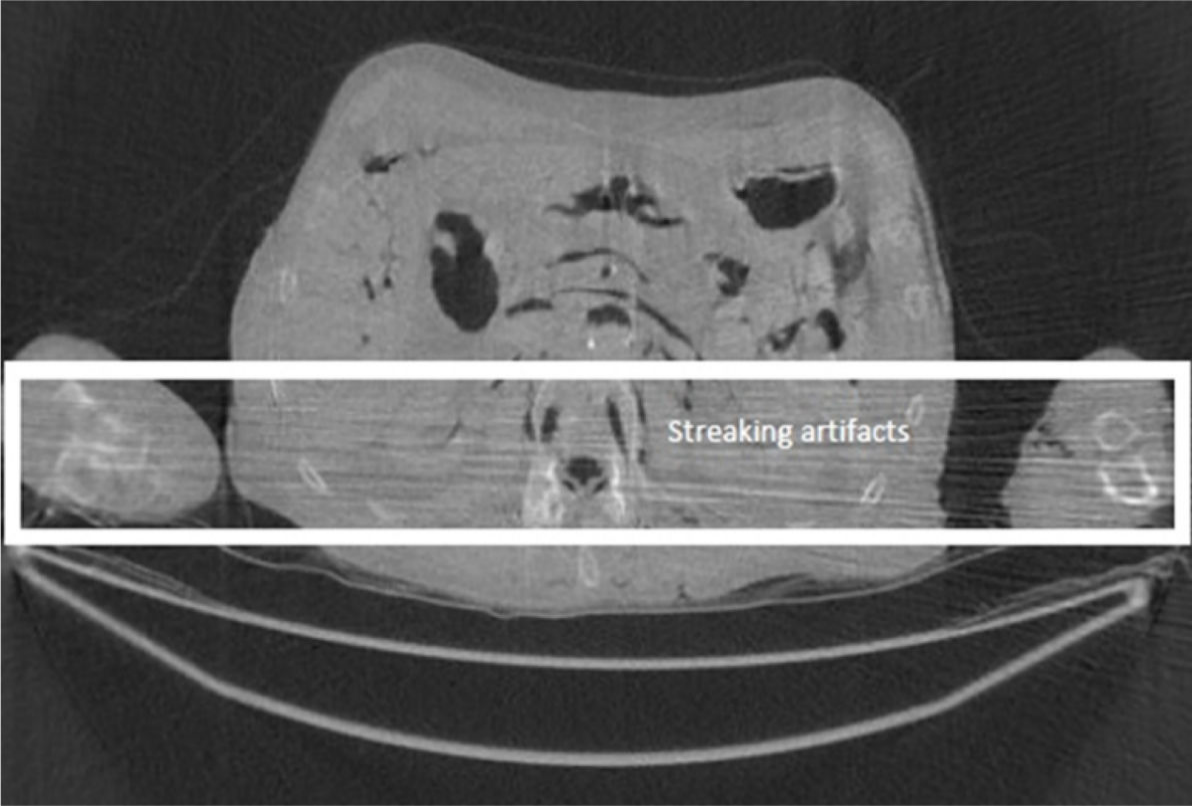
A CT image that suffers streaking line artifacts from arm to arm.
